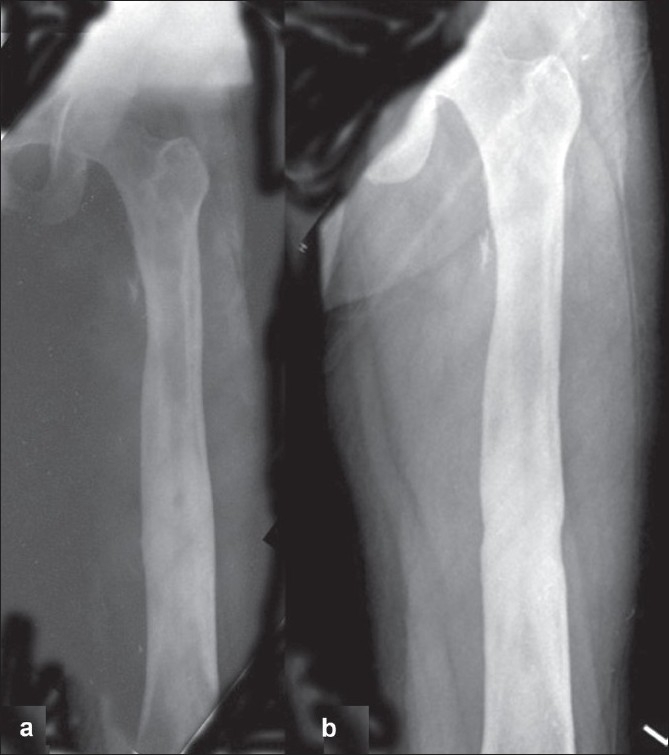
X-ray of left femur anteroposterior view showing (a) femoral cortical thickening. (b) Healed lesion after 30 months
